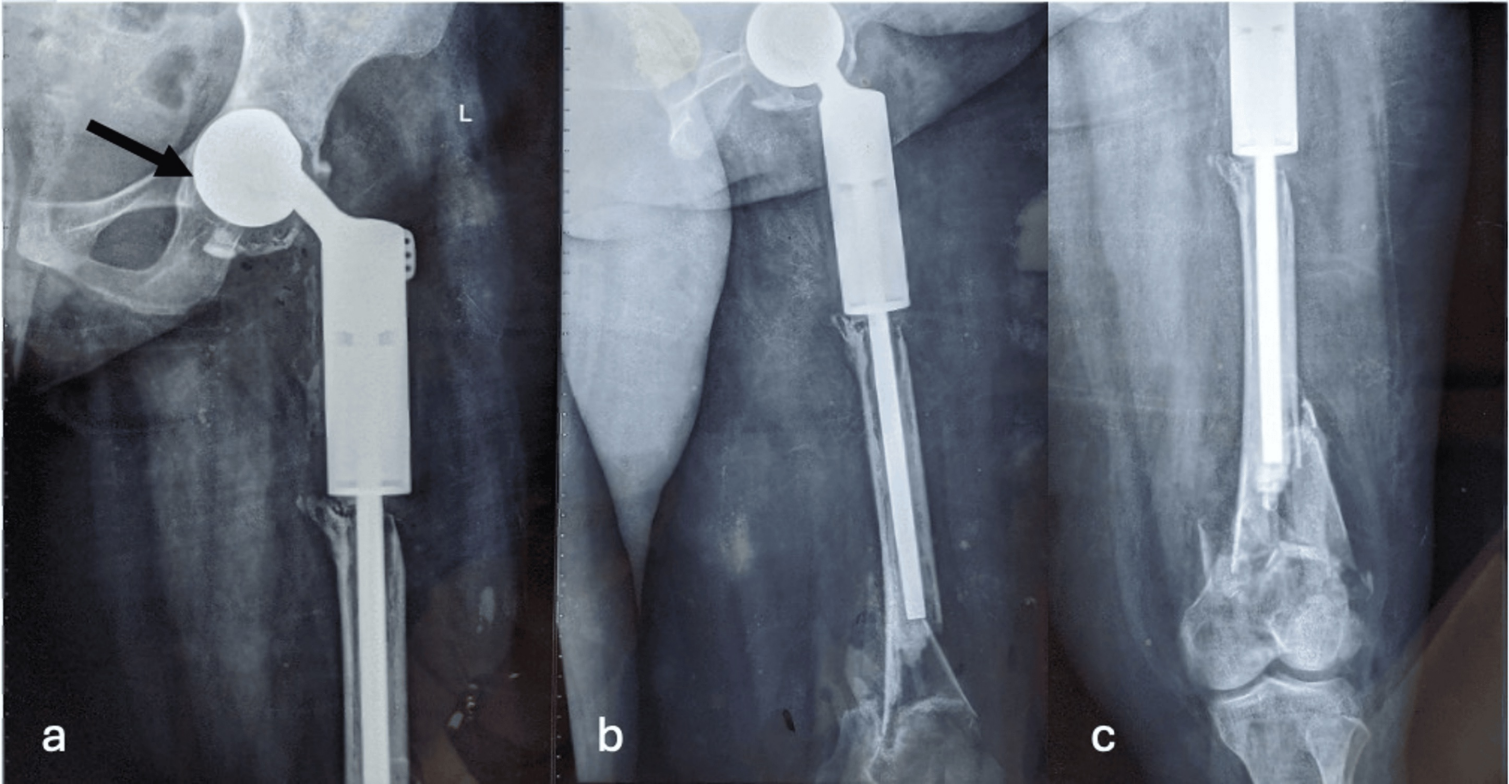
Preoperative X-ray of left side showing tumour megaprosthesis with comminuted periprosthetic distal femur fracture and acetabular component loosening a. X-ray(anteroposterior view) of the left hip and proximal femur, arrow shows acetabular loosening; b. X-ray(lateral view) showing the whole of the prosthesis and comminuted periprosthetic distal femur fracture; c. X-ray(anteroposterior view) of the distal femur showing comminuted periprosthetic distal femur fracture.
